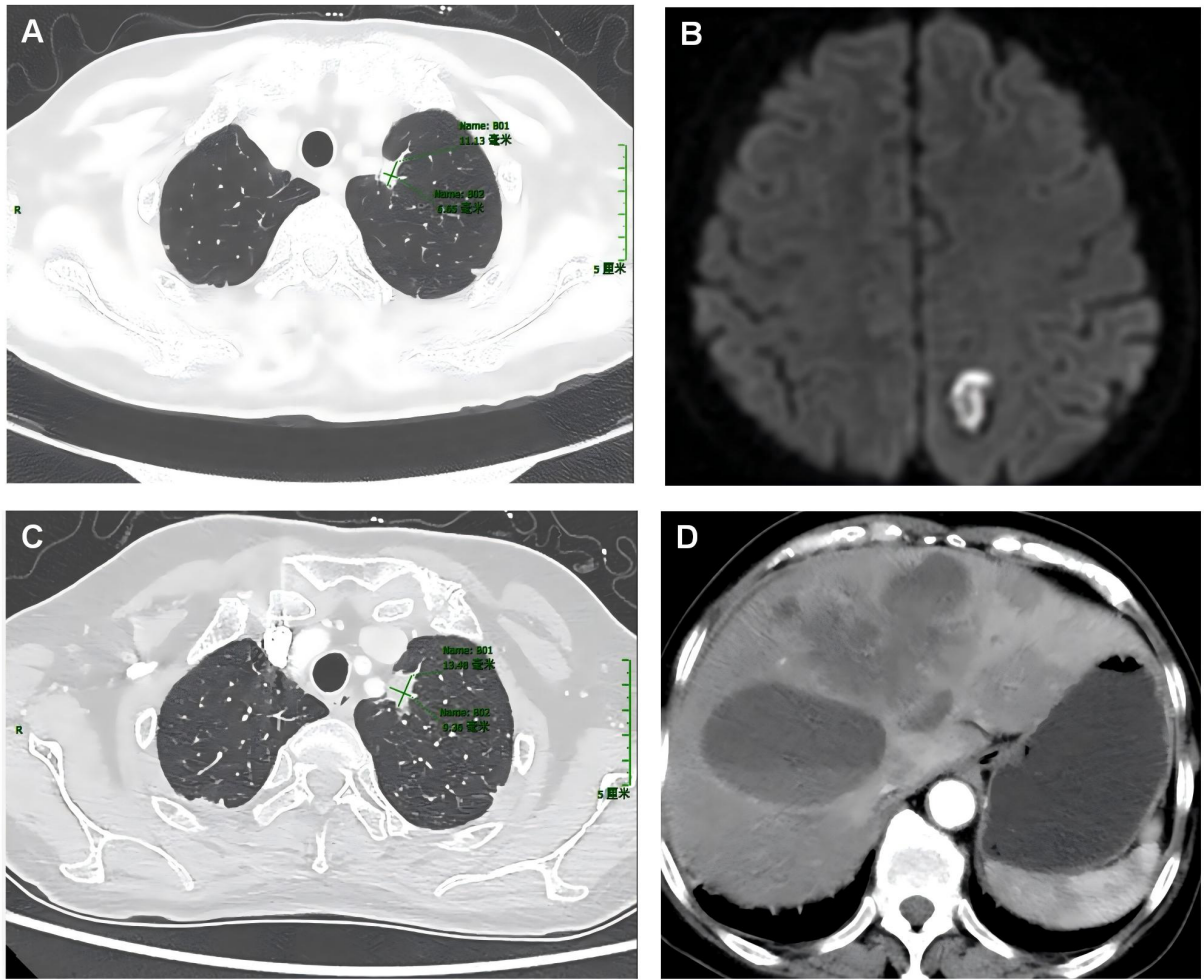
Plain computed tomography (CT) images of the lung and liver lesions, as well as the cranial magnetic resonance images of the first patient. The plain CT scan upon admission showed a mass in the lungs (A), whereas the magnetic resonance imaging of the brain indicated brain metastases (B). In September 2024, plain CT scans of the chest and liver showed lung lesions (C) and liver metastases (D).
